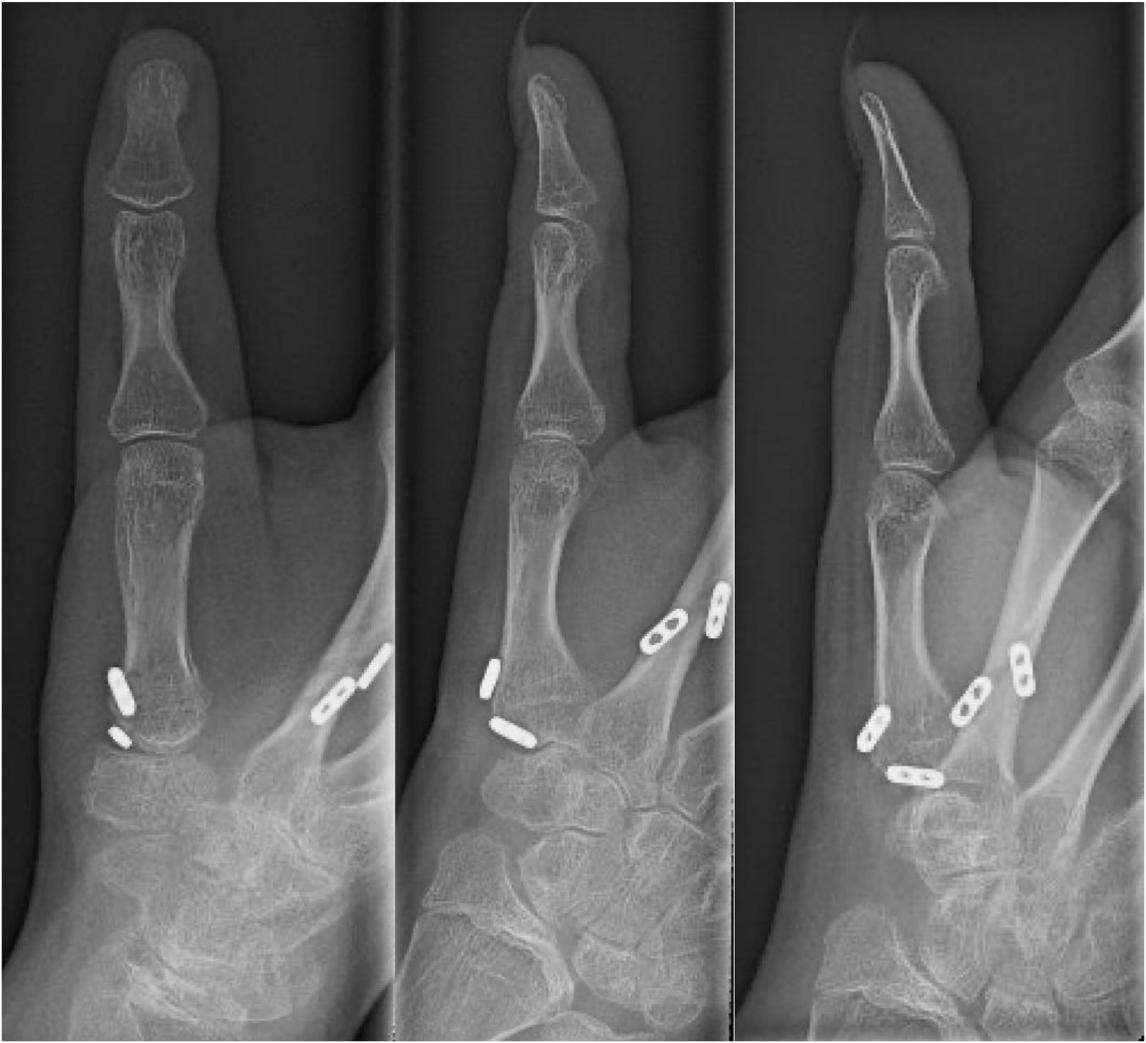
Fourteen-week postoperative posteroanterior, oblique and lateral radiographs of the right thumb demonstrating anatomic alignment of the thumb CMC joint with interval K-wire removal.
